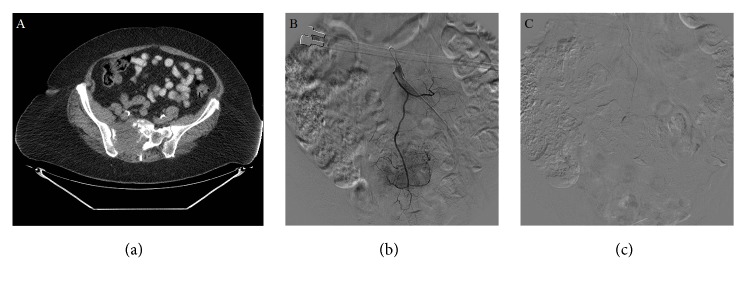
(a) Axial CT demonstrating osseous neoplasm in right sacroiliac wing in 72-year-old female patient. (b) Selective angiography of median sacral artery showing tumor blush and tumor vascularity. (c) Embolization of medical sacral artery with 300 micron PVA particles with diminished vascular supply.
